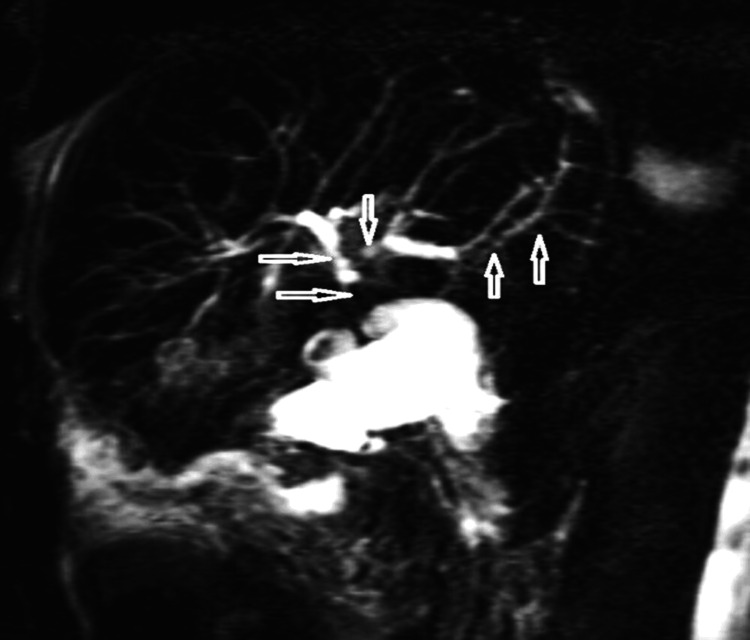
Selected image of T2 coronal HASTE radial MRCP showing sever stenosis of the hepatic duct and multiple areas of alternating IHD stenosis/dilatation (arrows) consistent with the diagnosis of PSC HASTE: half-Fourier acquisition single-shot turbo spin-echo; MRCP: magnetic resonance imaging cholangiopancreatography; IHD: ischemic heart disease; PSC: primary sclerosing cholangitis
